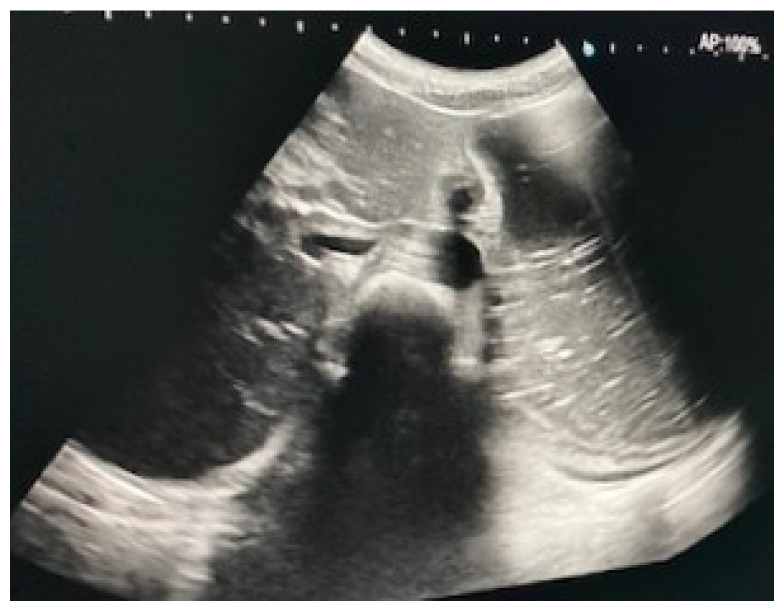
Abdominal ultrasound showing gastric distension.
